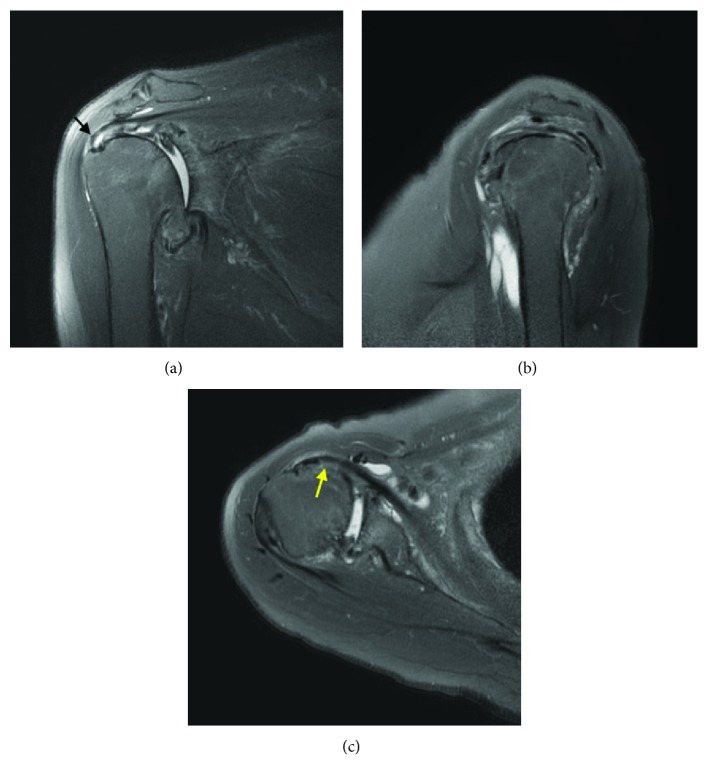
MRI T2-weighted of the right shoulder: coronal oblique view (a) showing a small size full thickness supraspinatus tear (black arrow), sagittal view (b), and axial view (c) showing tendinosis of the subscapularis muscle (yellow arrow).
